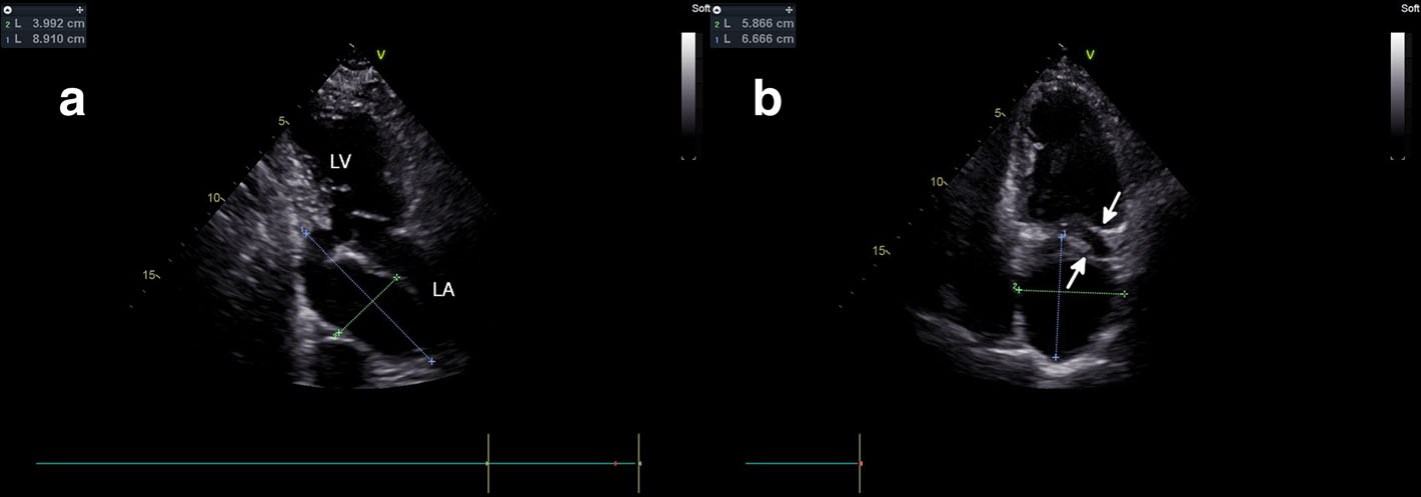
a Transthoracic echocardiographic apical 2-chamber view shows the left ventricular pseudoaneurysm(9x4cm) along the left atrium. LV (left ventricle), LA (left atrium). b Transthoracic apical 4-chamber view reveals the pseudoaneurysm(7x6cm), obviously compressed left atrium (white arrows)
